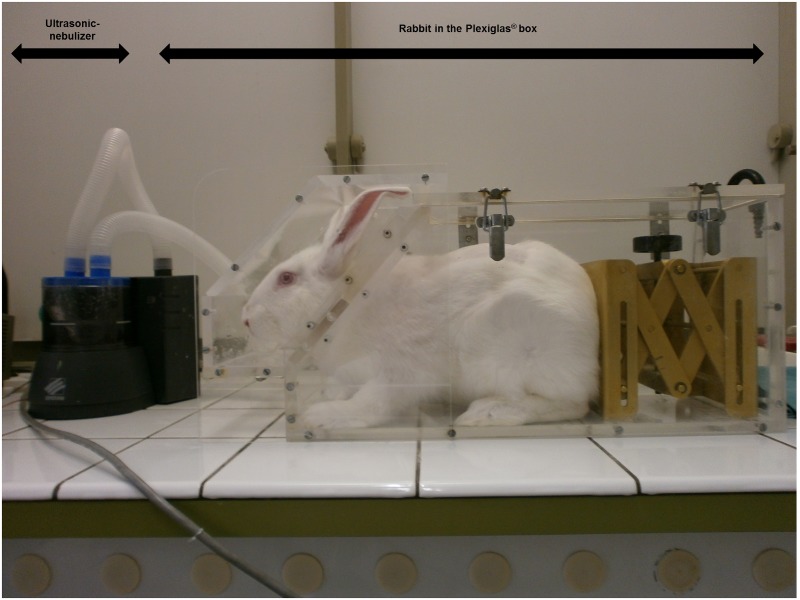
Rabbit exposed to an aerosol administrated in a closed Plexiglas box using an ultrasonic-nebulizer (SYST’AM, LS290). The box was conceived to allow the head of the rabbit to be maintained in front of the aerosol particles and drops cloud generated by the nebulizer. Aerosol sessions were performed 15–20 min daily from day 26 to day 28 either with ovalbumin (OVA rabbits) or with saline (control rabbits).
